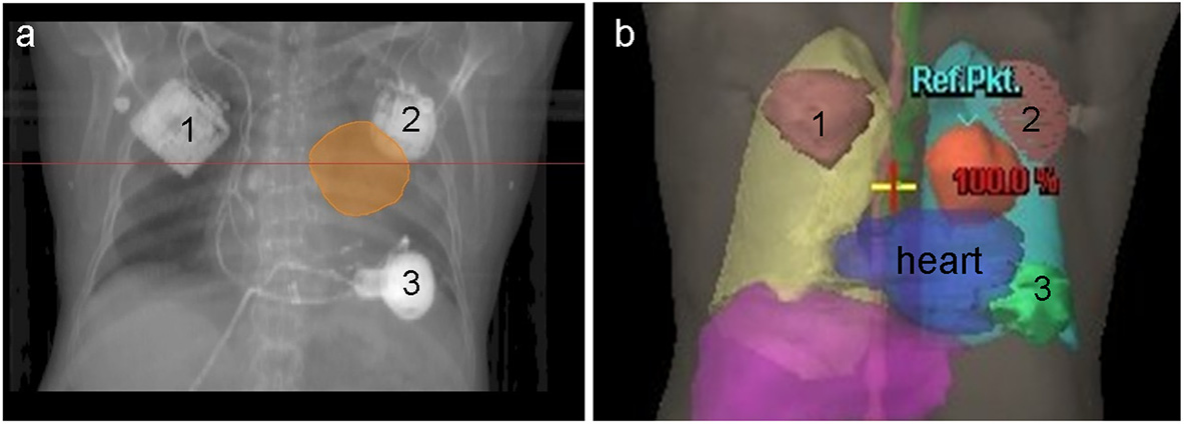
PTV in relation to implanted cardiac devices: a) breathing- and heart beat-guided definition of PTV on the basis of 4D List Mode PET/CT; b) partial localization of the ICD in the radiation field. 1- cardiac pacemaker, 2 – ICD, 3 – CPS device.
